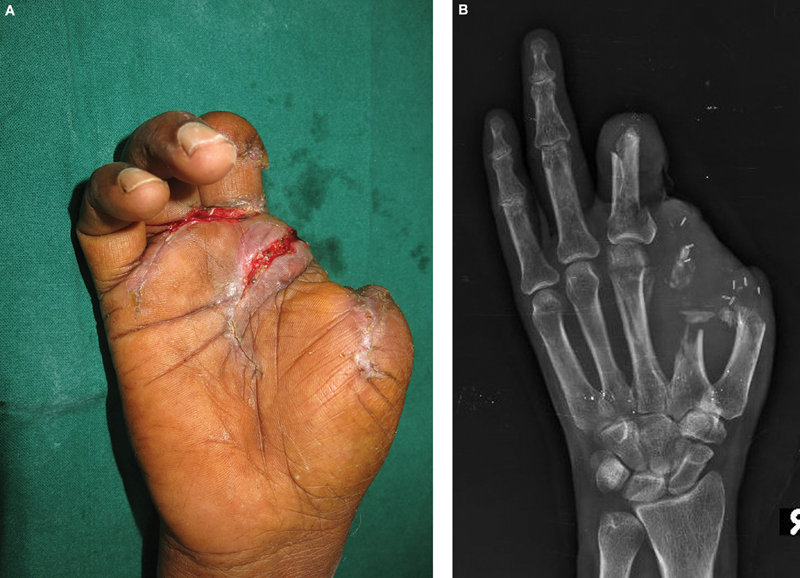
Presenting image and radiograph of the right hand. (
